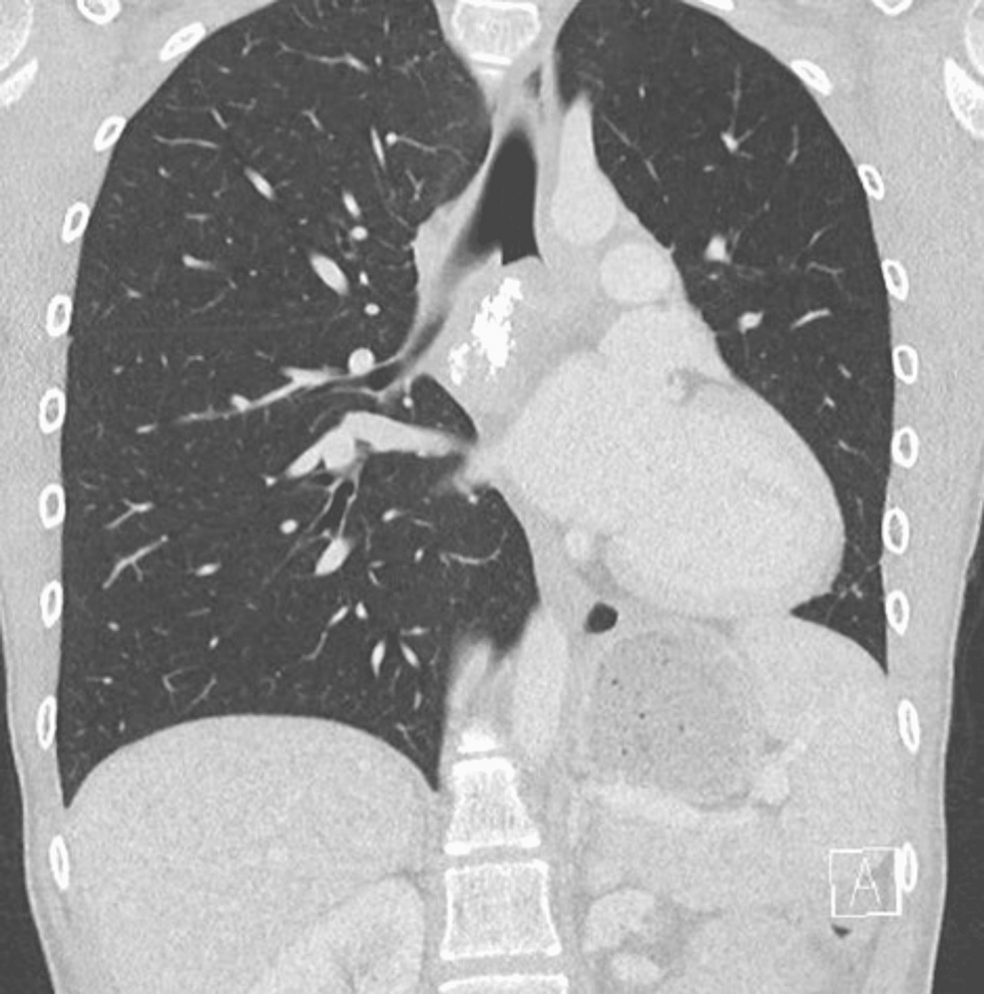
Chest CT coronal axial lung window views demonstrating subcarinal calcified lymph nodes with opacification and obstruction of the left mainstem bronchus. One of these calcifications is eroding the medial wall of the left mainstem bronchus. CT, computed tomography.
